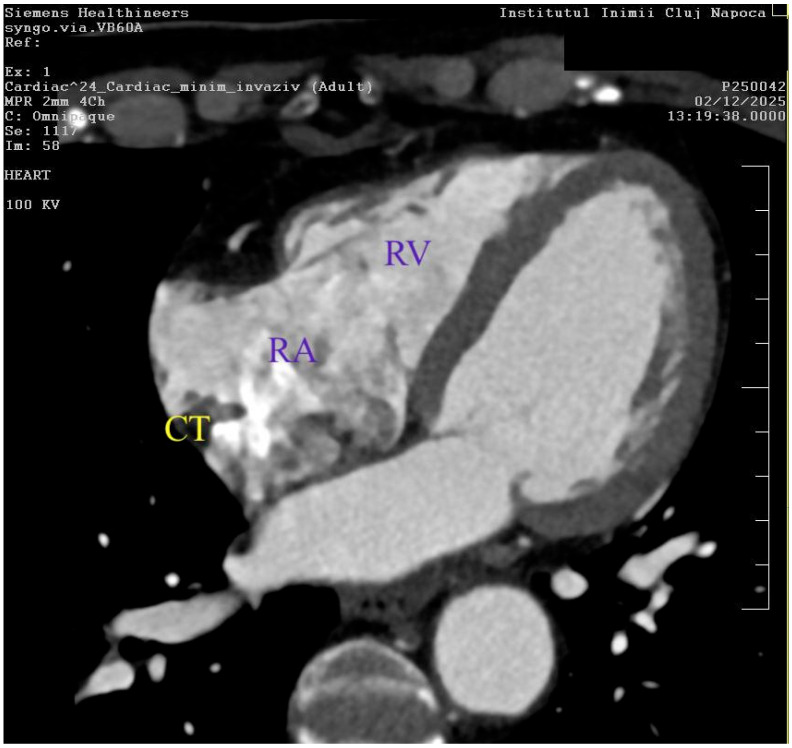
CCTA showing a filling defect within the right atrium, represented by the hypertrophied CT. CT: crista terminalis, RA: right atrium, RV: right ventricle.
